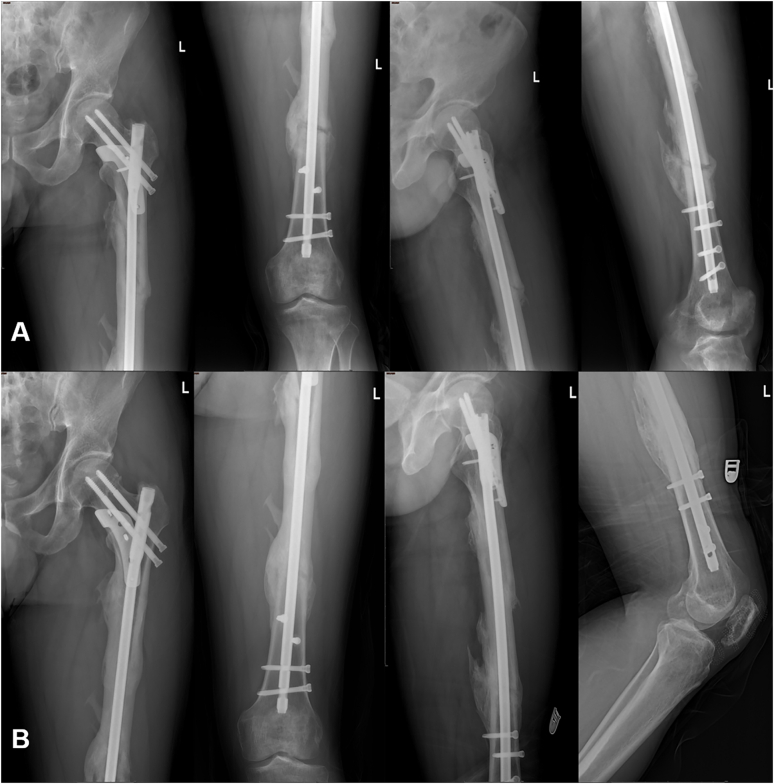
A: Radiographs at seven months after surgery. B: Radiographs at 17 months after surgery.
